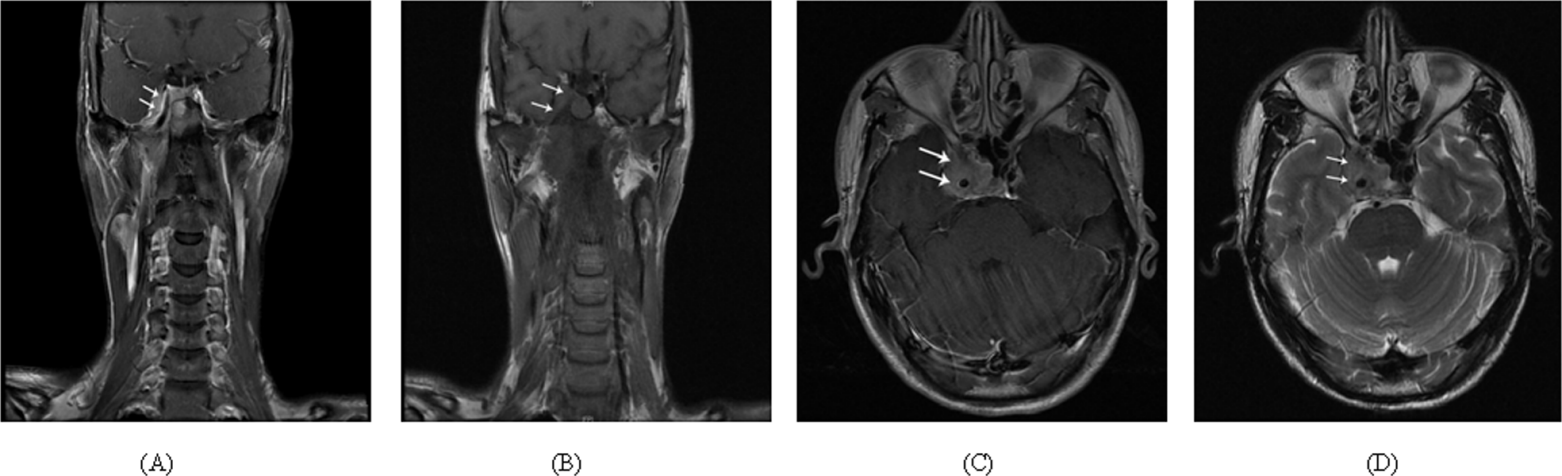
Representative appearance of cavernoussinus invasion (CSI) on multi-serial plane MRI scan images from an individual patient with NPC. (A) Enhanced coronal T1W fat-suppressed magnetic resonance (MR) image showing invasion of the right CS via the foramen lacerum (upward arrow). (B) Plain coronal T1W MR image of the same scanning plane as shown in (A). (C) The lesion is seen to extend to the right CS (arrow) and the orbital apex on the axial T1W MR image. (D) Axial T2W MR image of the same scanning plane as shown in (C).
